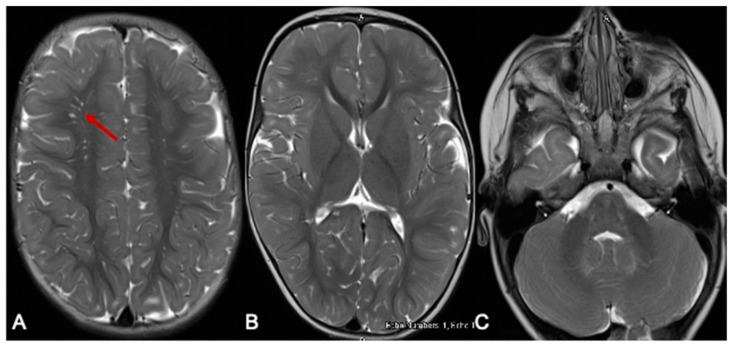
MRI of the brain 10 months later. The red arrow indicates the prominent perivascular spaces. From a follow-up MRI brain scan 10 months later, the T2-weighted axial images show significant improvement in myelination. Prominent perivascular spaces are also seen (A-arrow). There is resolution of the previously noted signal changes of basal ganglia and thalamus (B), and the brainstem and dentate nuclei (C).
